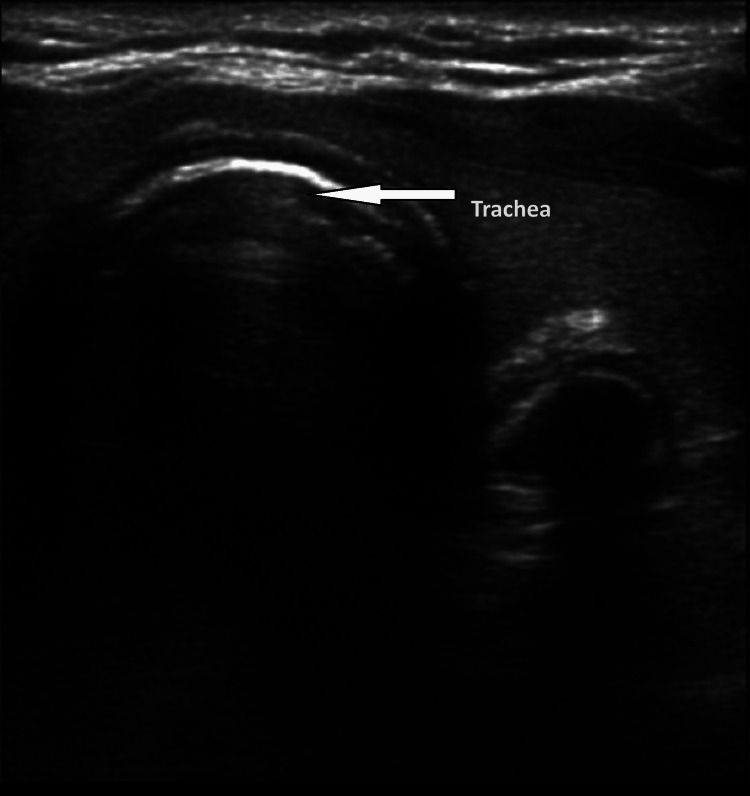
Ultrasonographic image of esophageal intubation showing double-tract sign
